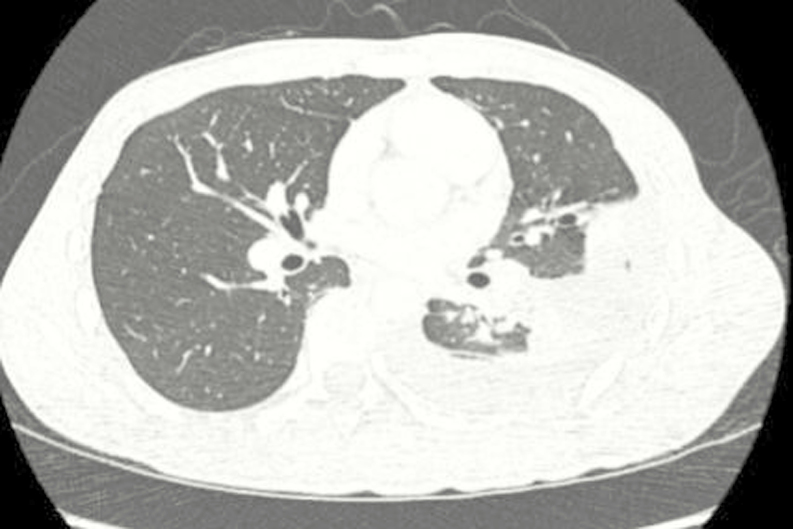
Chest CT demonstrated a moderately sized left-sided, loculated pleural effusion tracking along the major fissure without a rim of pleural enhancement to suggest empyema.
